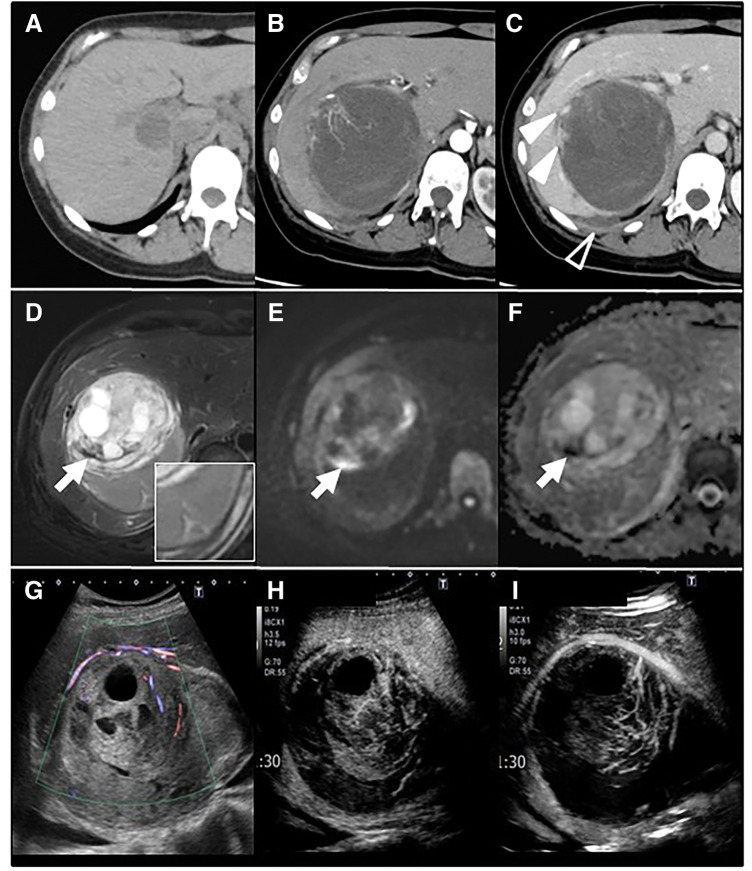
Multimodal imaging findings of the hepatic lesion. (A) A plain CT performed 2 years earlier revealed a 1.5 × 2 cm lesion in segment 6 of the liver. (B, C) A heterogeneous 11.5 cm low-density mass was identified, accompanied by high-attenuation extrahepatic fluid suggestive of bloody ascites (open arrowhead). Perinodular rich arterial vascularity was observed (B), and focal enhancement within the mass (solid arrowhead) persisted into the delayed phase (C). (D) T2-weighted MRI demonstrated a heterogeneous mass encapsulated by a hypointense rim. Very low–signal-intensity components (arrow) indicated hemosiderin deposition, corresponding to areas of diffusion restriction on (E) DWI and (F) ADC. (G) Contrast-enhanced ultrasonography revealed a basket-like pattern with several dilated feeding vessels within the septum. (H, I) In the early vascular phase, (I) the nodule showed no distinct enhancement compared with the surrounding liver parenchyma, followed by early washout. ADC, apparent diffusion coefficient; DWI, diffusion-weighted imaging
